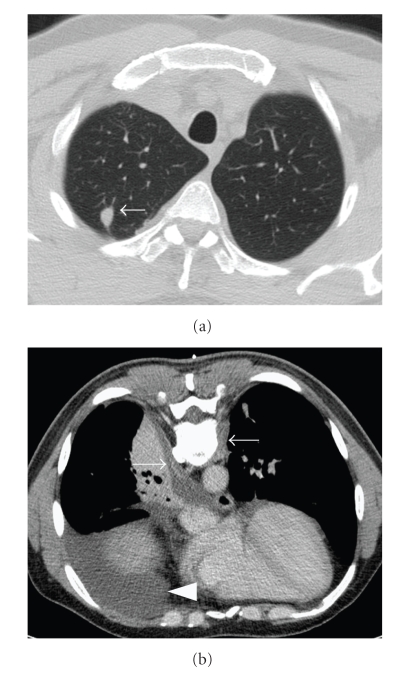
(a) CT scan revealing a nonspecific nodule in the right upper lobe (arrow). (b) Contrast enhanced CT in prone position shows right pleural effusion (arrowhead) and bilateral sub pleuralthickening (arrow) in the region of the 9th vertebrae.
